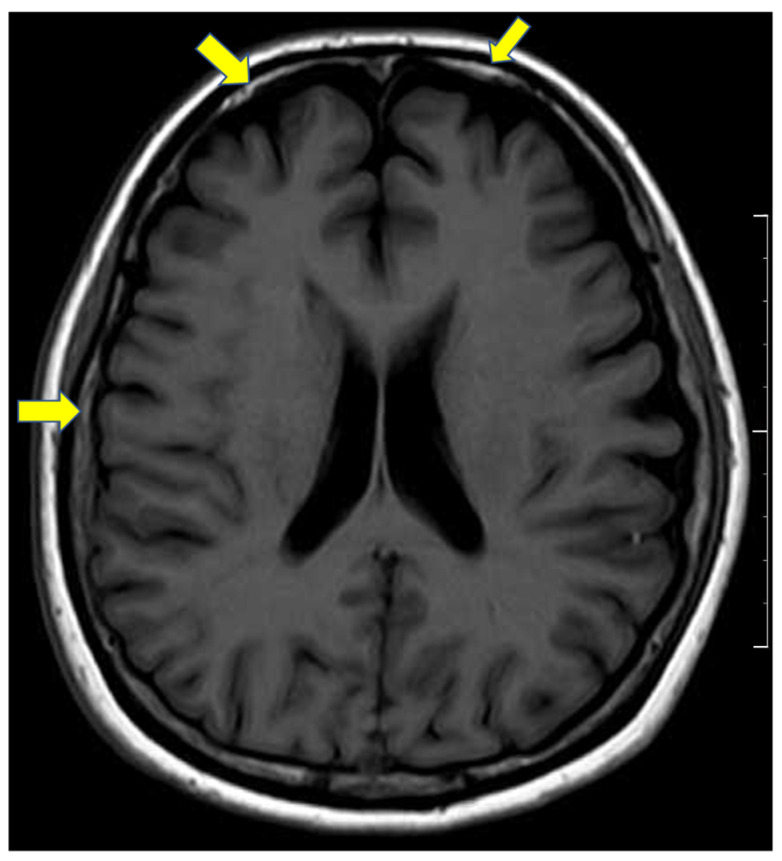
Magnetic resonance imaging (T1 series) showed continuous leptomeningeal enhancement (yellow arrows). Scale bar equals 10 cm.
